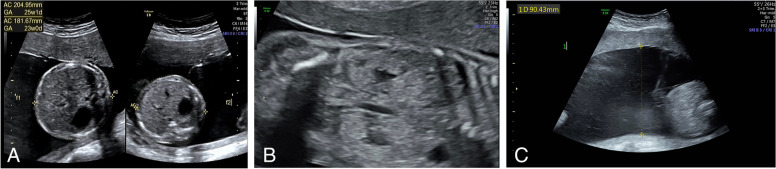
Ultrasound images at 22 + 2 gestational weeks. A, comparison of AC measurements between two foetuses (left: fetus A; Right: fetus B). B, hyperechoic renal cortex in foetus A. C, polyhydramnios in foetus A
